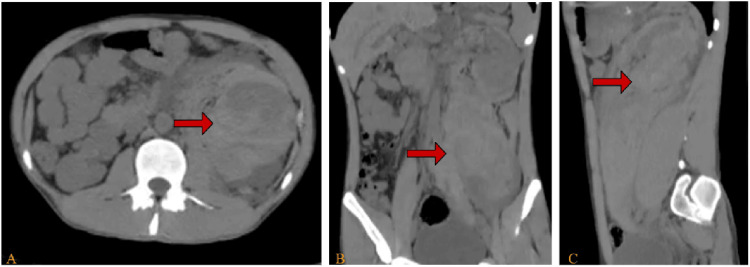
Axial (A), coronal (B) and sagittal (C) views of abdomen and pelvis CT without contrast at admission showing a huge left perinephric retroperitoneal hematoma measuring 20 x 12 x 4.5 cm
